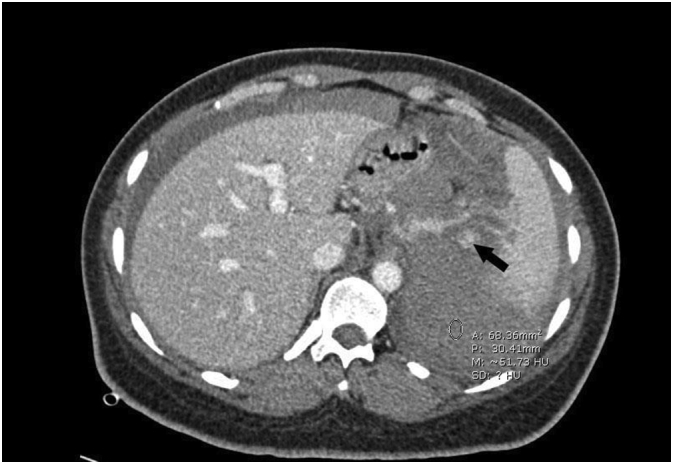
Shows abdominal CT angiogram venous phase with an arrow pointing at the site of the aneurysm and a circle indicating extensive hemoperitoneum (50 HU), no active contrast extravasation could be seen.
